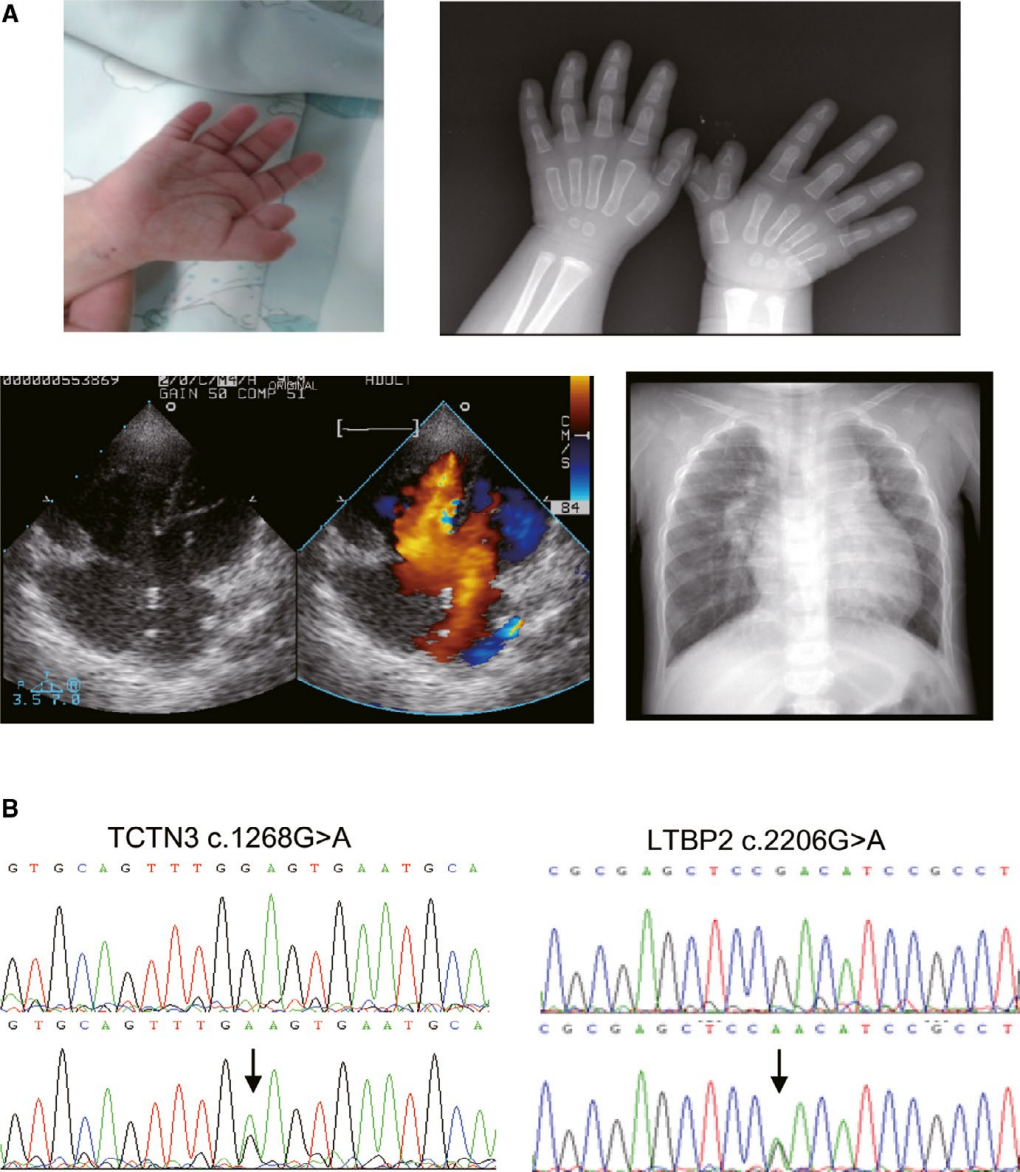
Typical phenotypes of the patient and potential pathogenic mutations. (A) Clinical features of the patient with both congenital heart defects and polydactyly. Atrioventricular septal defect (AVSD) was diagnosed by echocardiography; The X‐ray shows the enlarged heart shadow and the polydactyly. (B) Chromatograms of the two heterozygous mutations in the study. Top panels show wild type, and bottom panels show heterozygous mutations. Mutations are marked with arrows
